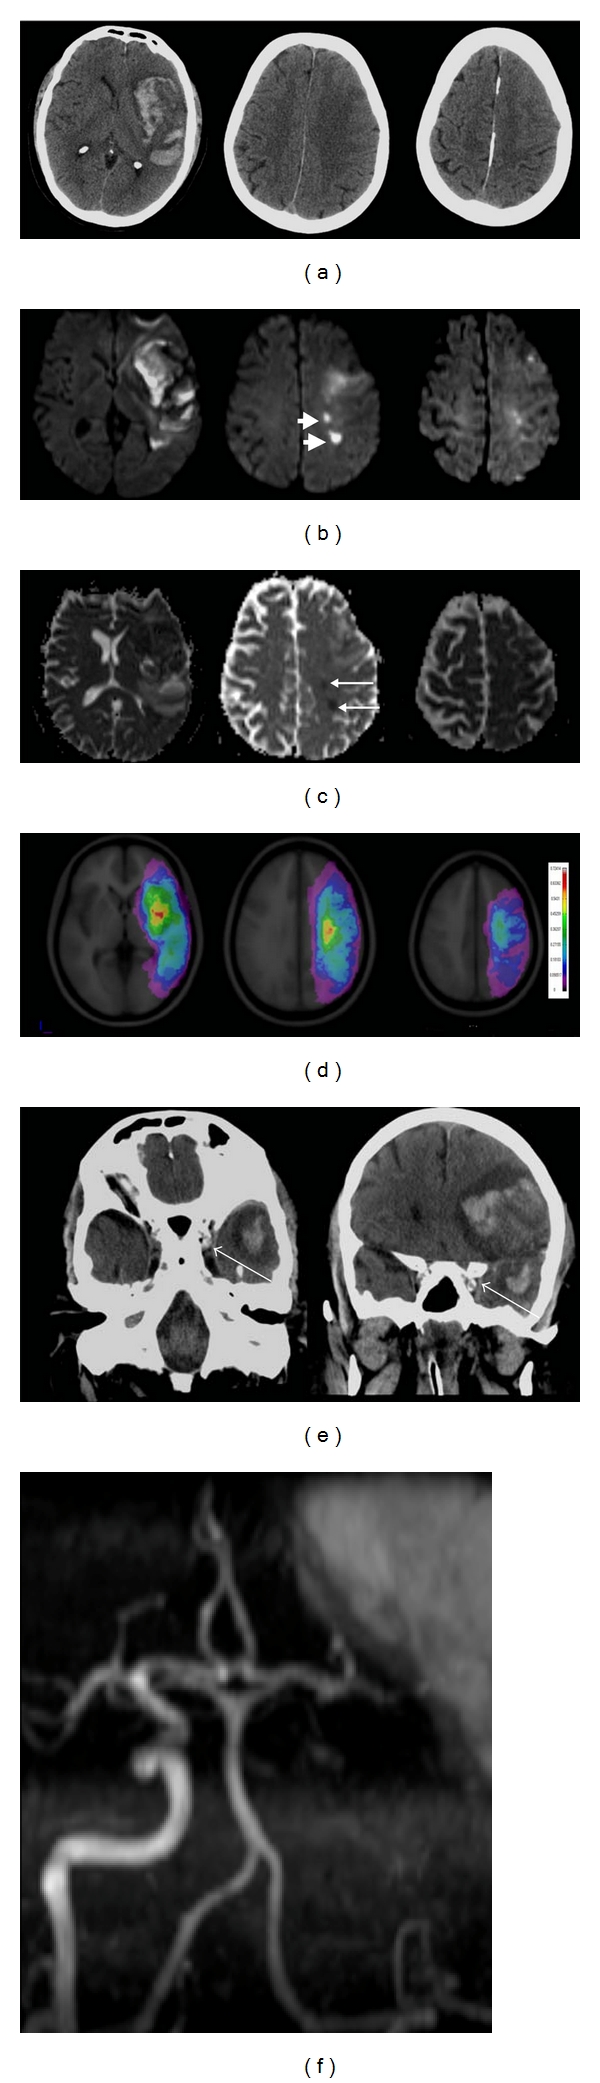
54-year-old man with a history of idiopathic thrombocytopenic purpura presented with acute coronary syndrome. He developed a dense right hemiparesis overnight. (a) Initial axial unenhanced CT images show a large left “fronto-temporal hemorrhage”, initially thought to be secondary to his low platelet count of 20. Stage IIH c2 [1]. (b) Axial diffusion weighted MR images reveal areas of restricted diffusion remote from the area of hemorrhage (arrow heads). (c) The areas of diffusion weighted abnormality have low apparent diffusion coefficient values (arrows). (d) Digital probabilistic maps of middle cerebral artery territory infarcts show both the infarcts and hemorrhage lie within the middle cerebral artery territory. (e) Coronal unenhanced images from the original CT show a dense left internal carotid artery in the cavernous sinus (thin arrow). (f) MR angiogram confirms occlusion of the left internal carotid middle cerebral arteries. The overall picture suggests that the stroke is a HI.
